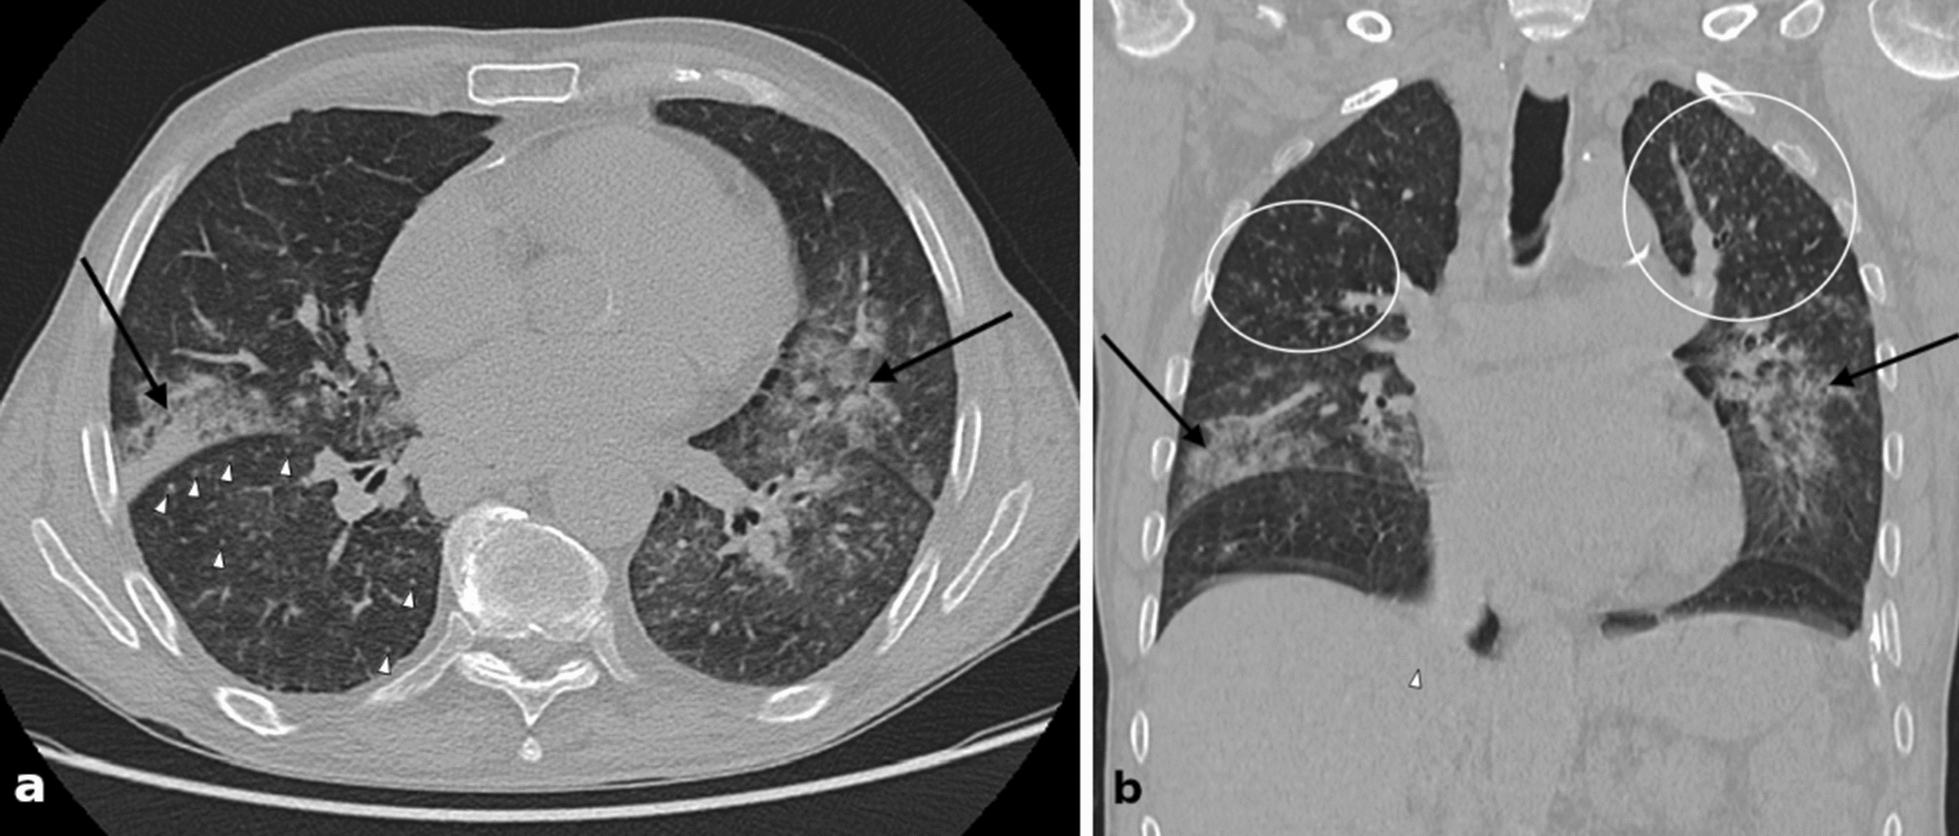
Chest computed tomography (axial [a] and coronal [b] non-contrast enhanced source images) from day 2 showing bilateral patchy consolidations (black arrows) and subtle micronodules, which are difficult to distinguish from small pulmonary vessels (white arrows and white ovals)
